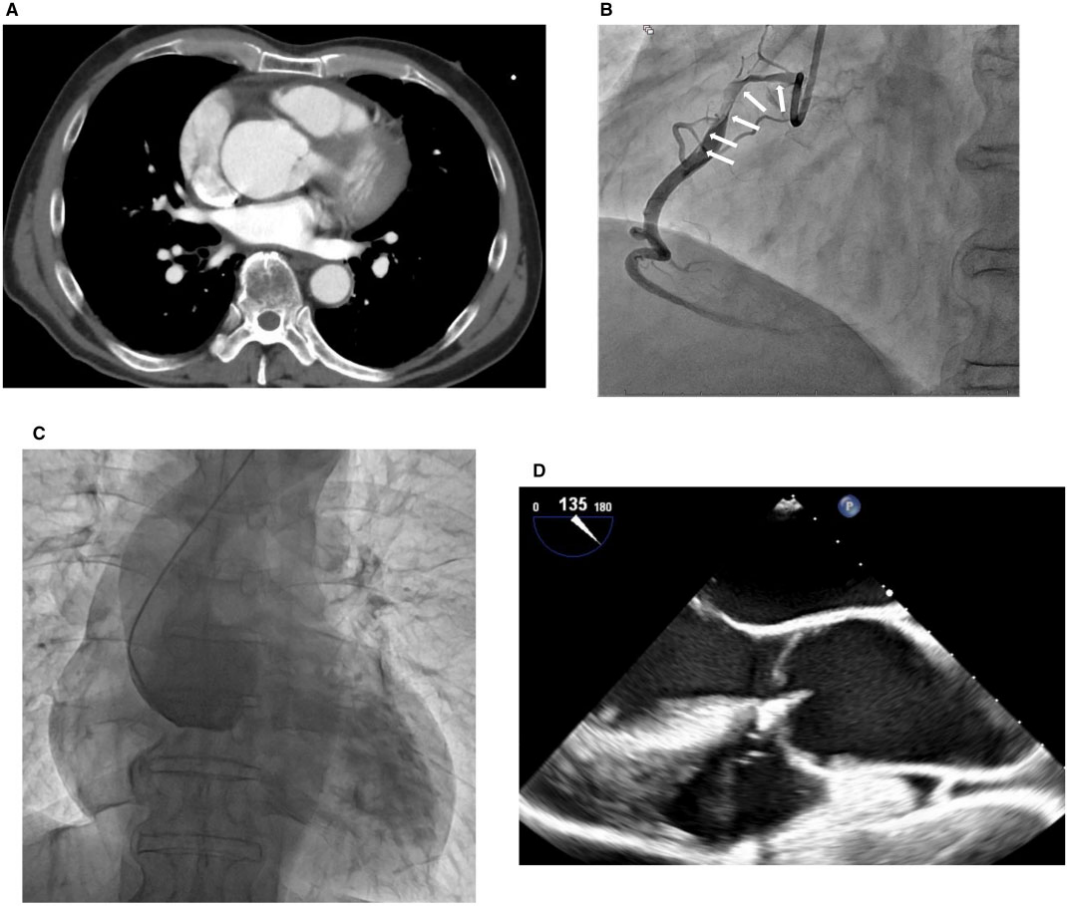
Enhanced computed tomography, coronary angiography and transoesophageal echocardiography findings. (A) Contrast-enhanced computed tomography images did not indicate an obvious intimal flap in the aortic root. (B) Coronary angiography revealing total occlusion of the right coronary ostium with an intimal tear (arrows). (C) Aortic root angiography revealing no flap. (D) Transoesophageal echocardiography revealing no intimal flap of the aortic root and the presence of prolapse of the non-coronary cusp.
